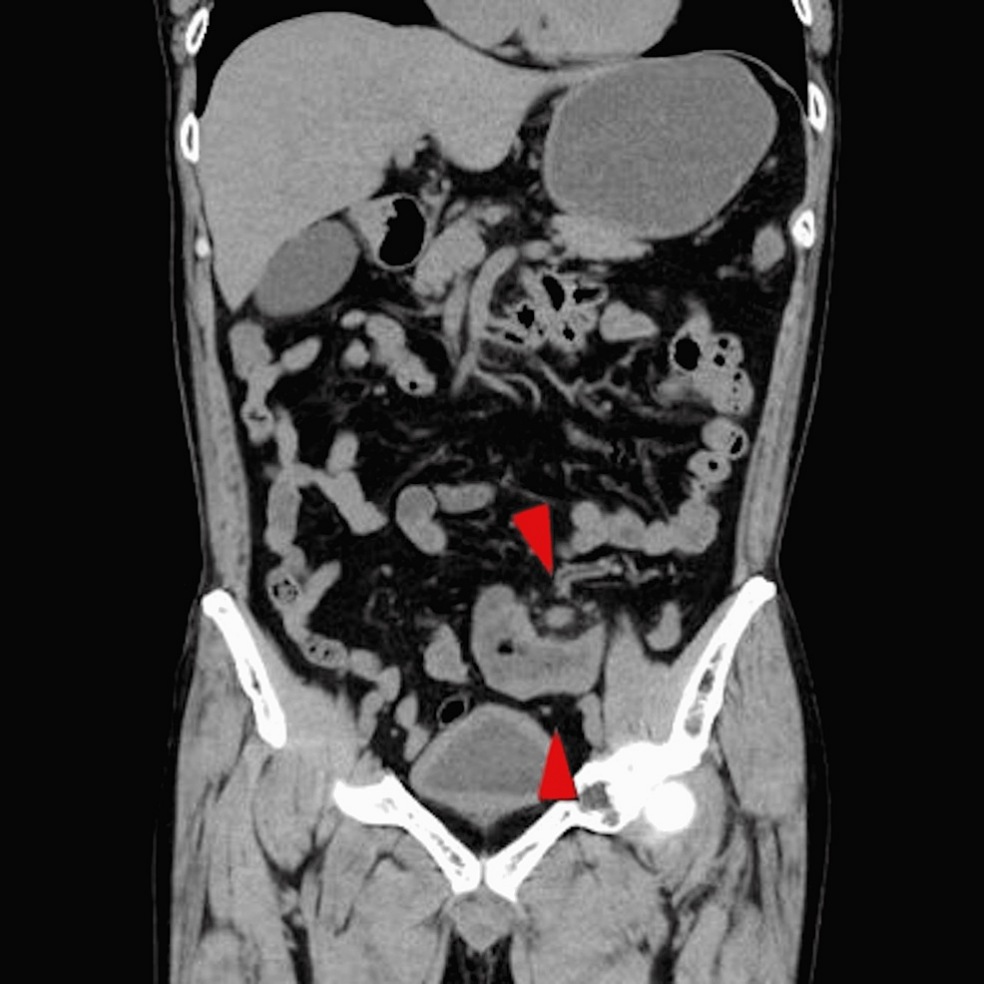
Non-contrast abdominal CT (coronal plane) showing edematous thickening of the wall extending the left side of the transverse colon to the sigmoid colon, enlarged lymph nodes, and increased density in the fatty tissue of the surrounding mesentery (arrows)
